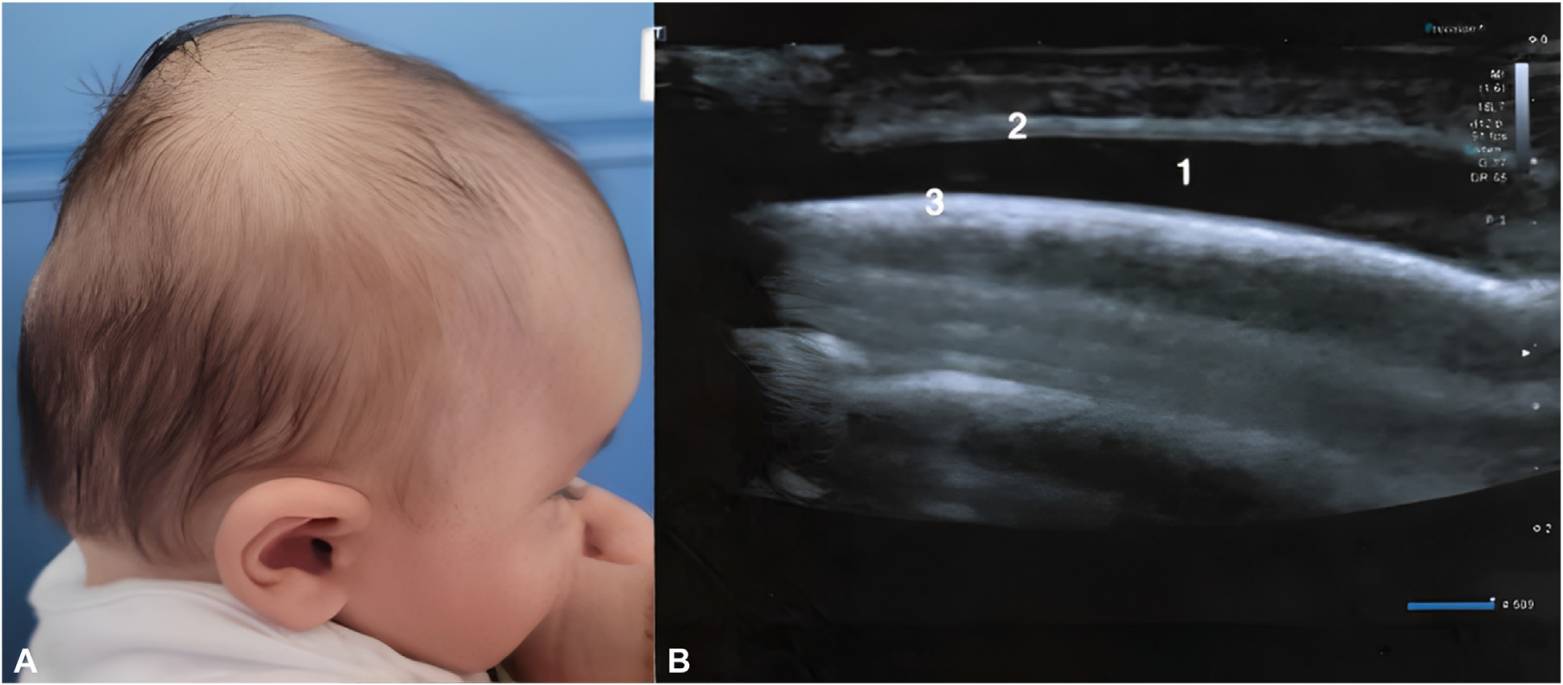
A, 7 × 5 centimeters parietal scalp swelling; B, ultrasound of this subgaleal collection (1: subaneurotic fluid collection; 2: galea aponeorotica; 3: periosteum of the parietal bone).
